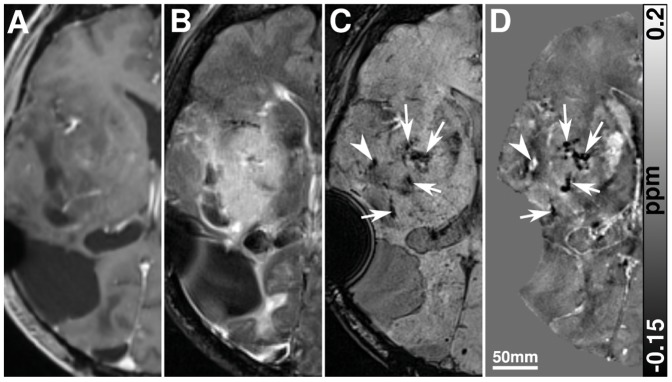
Images of a 49 year-old man with a glioblastoma with an oligodendroglial component after radiochemotherapy. The contrast-enhanced T1-w and FLAIR images are presented in (a) and (b), respectively. SBS, indicated by the arrow and arrow head, are discernable on the SW image (c). The corresponding susceptibility map in (d) clearly suggests that these SBS are likely produced by calcium deposits (arrow) and blood products (arrow head).
